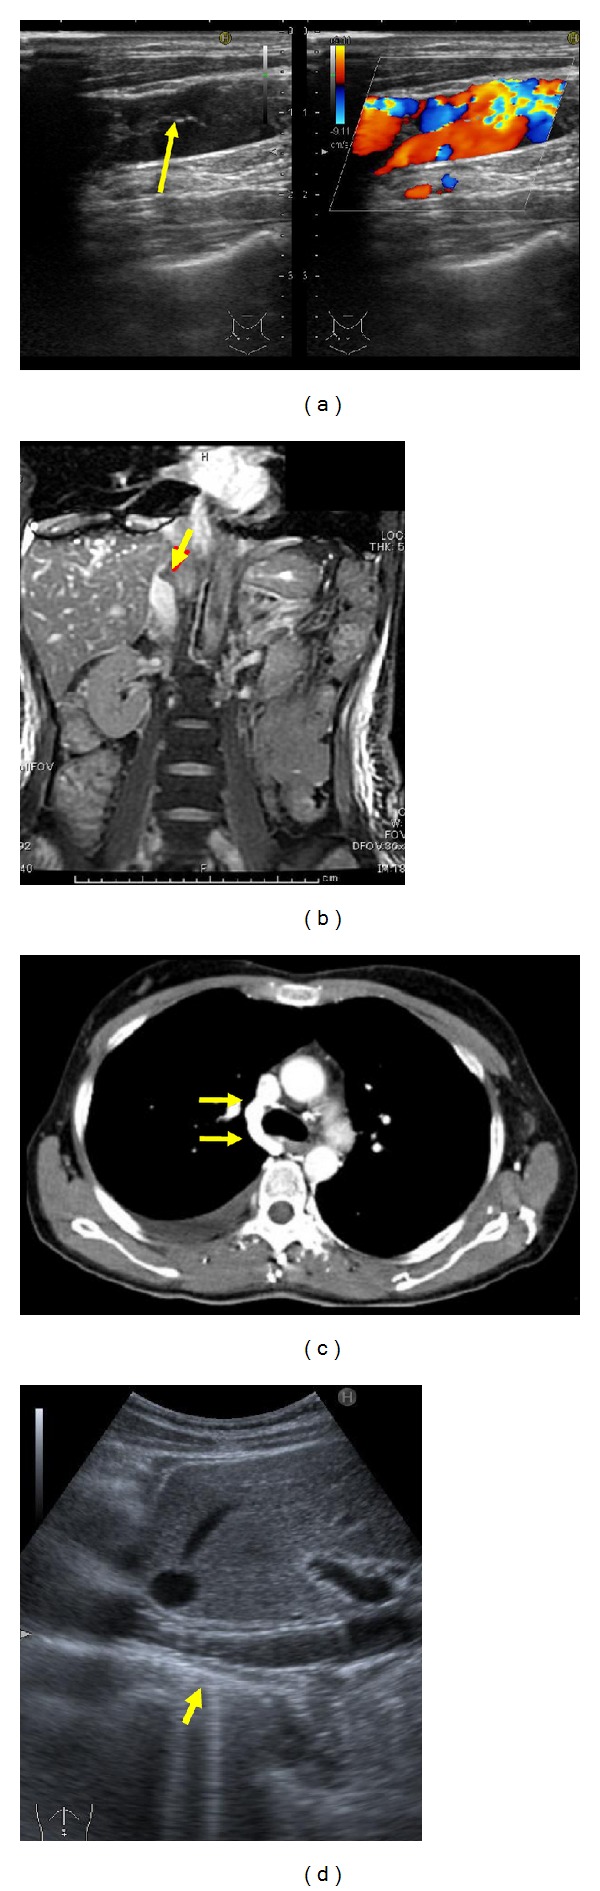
Imaging studies. (a) Carotid ultrasonography shows a thrombus of the right internal jugular vein indicated by the arrow. Blood flow in the right internal jugular vein was maintained. (b) Abdominal MRI first imaging employing steady state acuisition (FIESTA) reveals a high-intensity signal emanating from the intrahepatic portion of the inferior vena cava (arrow), which suggested thrombus. (c) Thoracic CT during injection of contrast medium reveals right pleural effusion and dilatation of an azygos vein at the arrows. (d) Abdominal Doppler ultrasonography shows complete patency (arrow) and no ectopia of the stent. This image was acquired 16 months after the patient was admitted.
